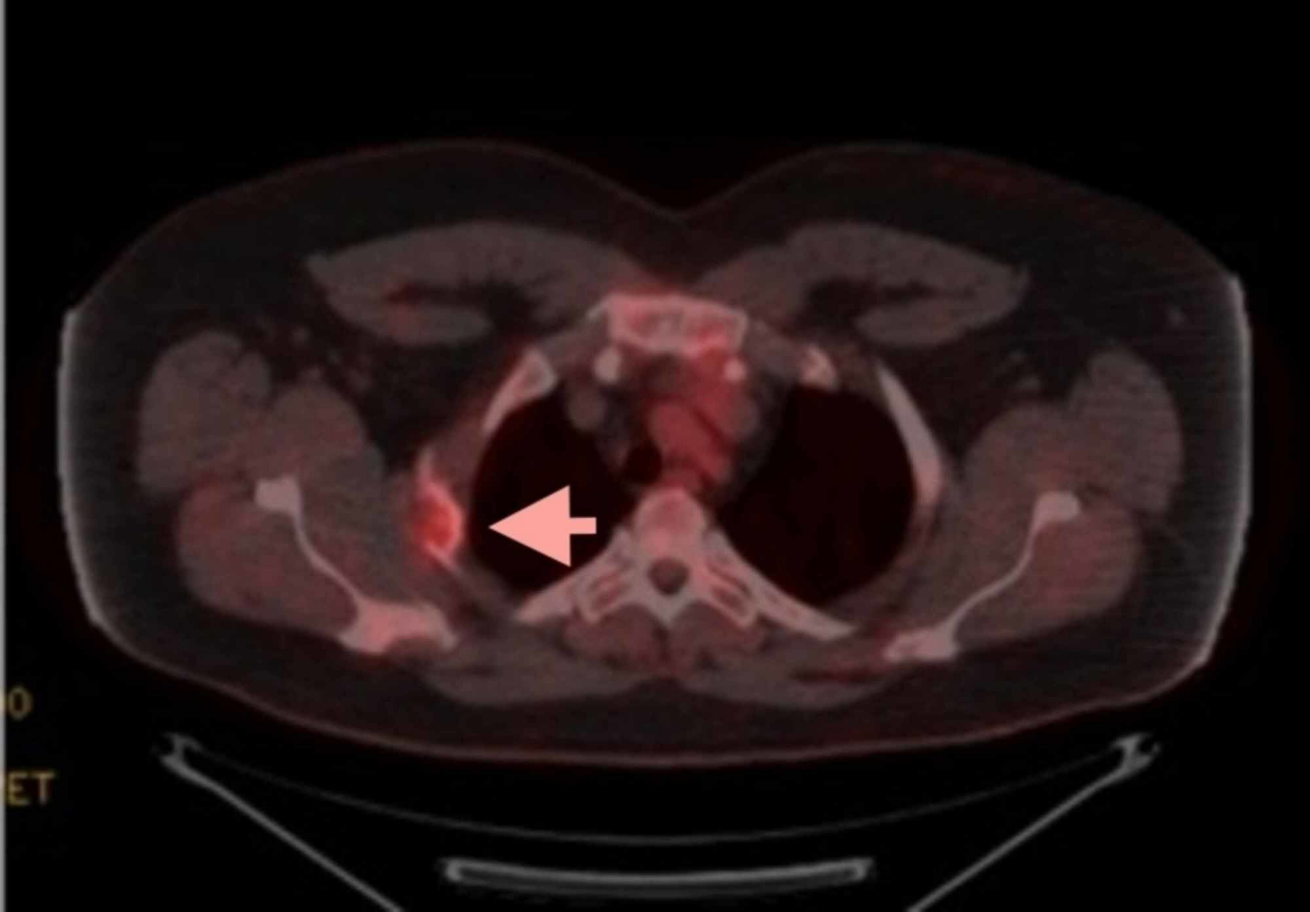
Positron emission tomography (PET) scan demonstrating F-18 fluorodeoxyglucose (FDG)-avid lytic lesion in the second right rib
